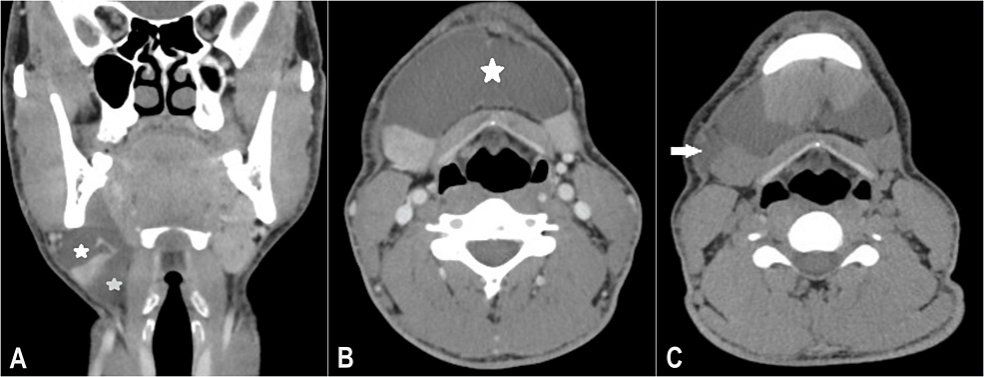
(A) Coronal CT image showing hypodense fluid collection above (white star) and below (blue star) the mylohyoid muscle. (B) Axial CT image with contrast, showing well-defined swelling in the right and left submandibular region (white star). (C) Axial CT image showing “tail sign” phenomenon (white arrow)
